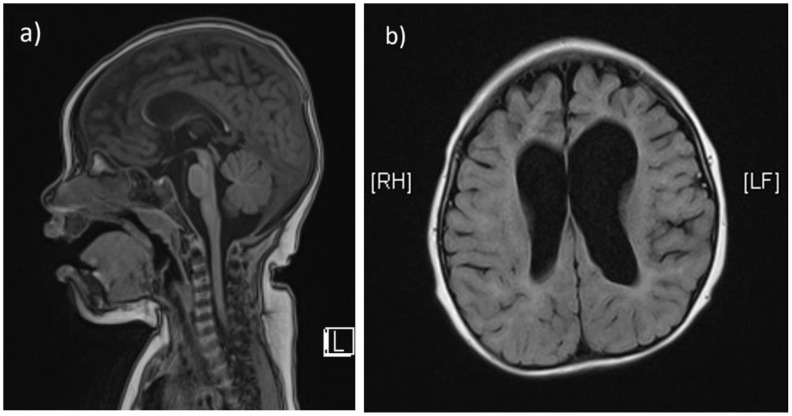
The T1-weighted, sagittal section of brain MRI from patient 2 also shows marked thinning of the corpus callosum (a). The T2-weighted, axial section shows prominent enlargement of cerebral cortical sulci and ventricles (b).
